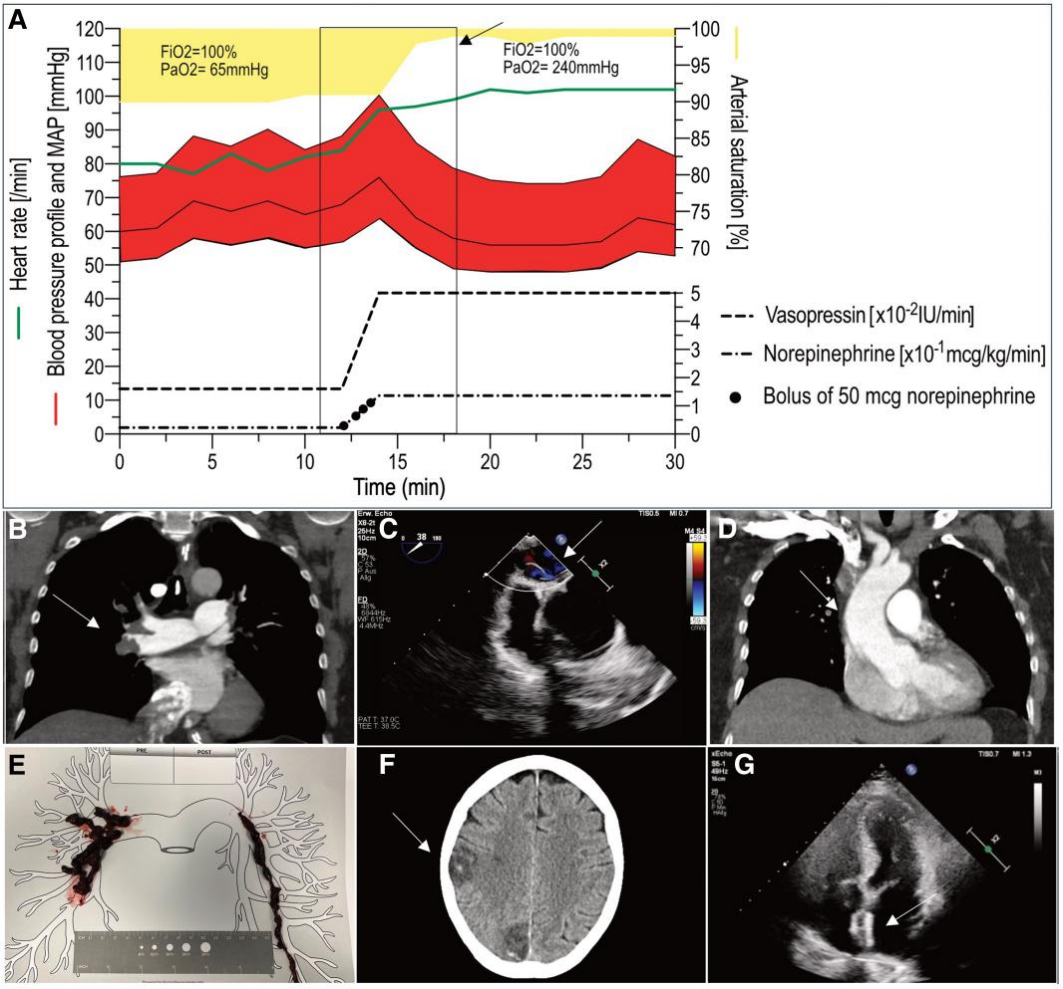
Two days after knee surgery, a 61-year-old female patient developed acute dyspnoea followed by hypoxaemic respiratory failure. The patient had no medical history. A week before, she had been able to cycle to work. A CT scan revealed bilateral central pulmonary artery emboli despite the administration of thromboprophylaxis (*Panel B*). In critical hypoxaemic failure despite non-invasive ventilation (NIV), bronchoscopic intubation was performed in upright position through the oronasal NIV mask. After the upper body was lowered, there was a sudden haemodynamic collapse accompanied by a significant improvement in oxygen saturation (*Panel A*). With persistent hypoxaemia, a right-to-left atrial shunt was suspected. In line, transthoracic echo revealed a jet on colour Doppler. Transoesophageal echocardiography provided further information on the morphology, showing a patent foramen ovale (PFO) (*Panel C*). The thin, rather mobile atrial septum was displaced to the left atrium resulting from high right atrial pressure. When pulmonary embolism initially increased pressures in pulmonary circulation, the right-to-left shunt most probably had prevented the right heart from acute failure. Lowering the upper body in the presence of a PFO as well as aortic elongation (*Panel D*) reduces the right-to-left shunt volume in orthodeoxia syndrome. In our case, reduced right-to-left shunt volume improved oxygenation but unmasked the fulminant obstructive shock. As progressive shock could not be stabilized by conventional therapy, veno-arterial extracorporeal membrane oxygenation (VA-ECMO) was established. Ischaemic stroke within previous six months was a contraindication for systemic fibrinolysis. Due to acute haemodynamic instability, interventional aspiration thrombectomy was performed and removed a large amount of thrombus material (*Panel E*). After further clinical stabilization, the ECMO could be removed after 5 days. On Day 8, the patient was extubated. Patent foramen ovale was most likely causal for multi-temporal, subacute, and older cerebral ischaemic insults, which were detected by CT (*Panel F*). After interdisciplinary assessment and shared decision-making with patients’ relatives, early transcatheter closure was performed (*Panel G*). The coagulation disorder assessment did not reveal thrombophilia. After neurological rehabilitation, the patient was discharged with oral anticoagulation and is now an active part in her family’s daily life again.
